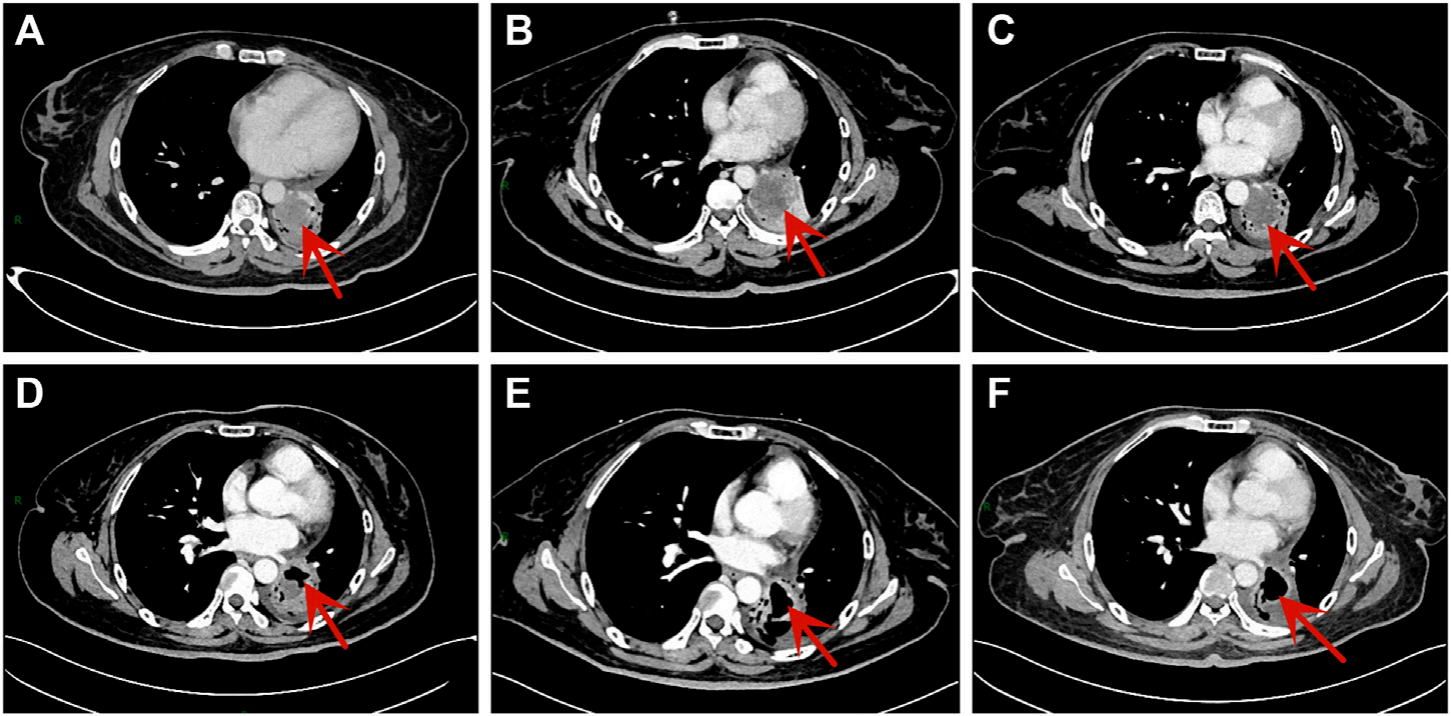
Chest CT scans of the patient. (A) The scan was performed after second-line treatment. (B) The scan was performed after third-line treatment. (C) The scan was performed after taking anlotinib for one cycle. (D)The scan showed tumor cavity formation after taking anlotinib for six cycles. (E) The scan showed tumor cavity was larger after taking anlotinib for eight cycles. (F) The tumor cavity didn’t change significantly after taking anlotinib for 10 cycles.
